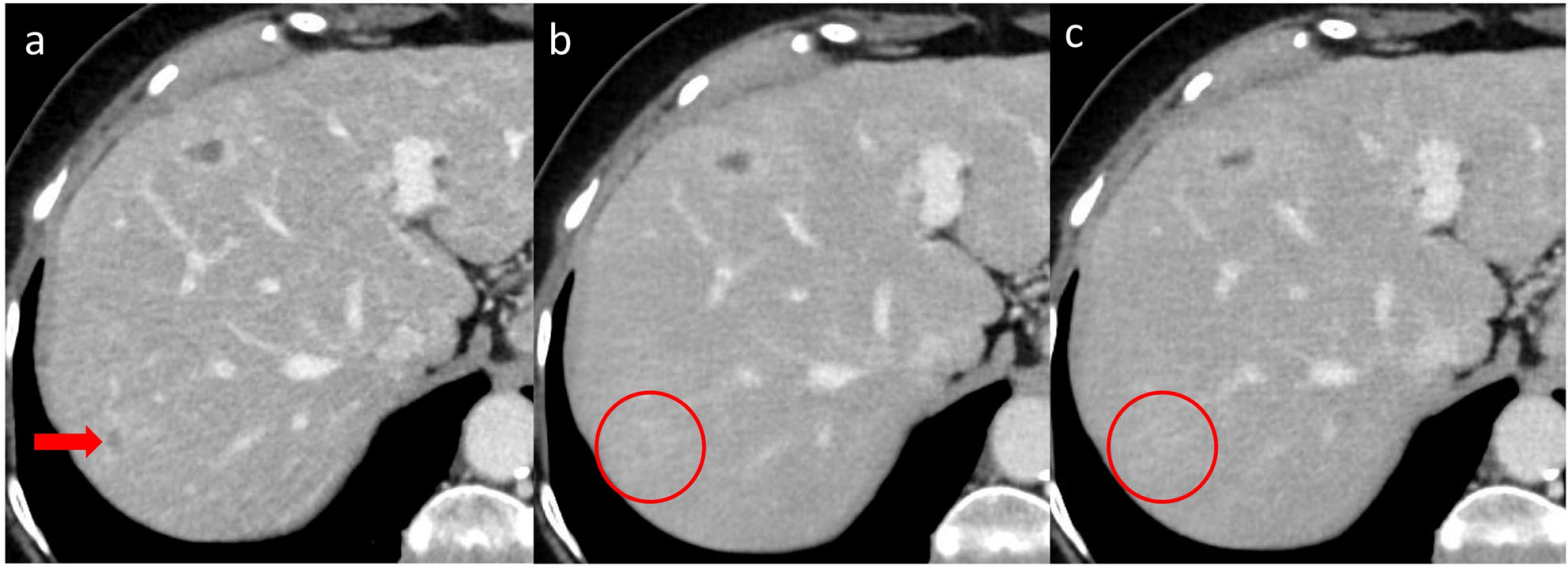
Axial contrast-enhanced CT images obtained with IR at standard-dose CT (a), DLR at medium-dose CT (b), and DLR at low-dose CT (c). A small, 9 mm hypodense liver lesion was detected by both readers at standard-dose IR (red arrow) but missed by both readers at lower dose levels with DLR (red circle). Reprinted, with permission, from reference [21]
